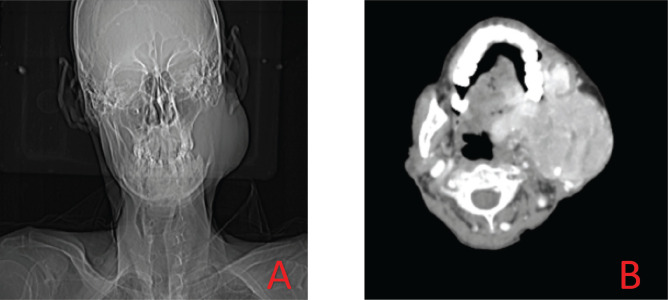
(a and b): CT images of tumour.
